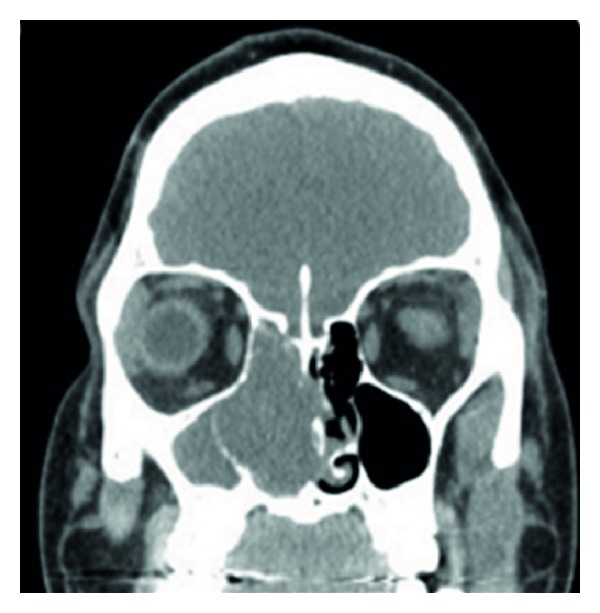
A noncontrast head CT revealing an LCH extending from the right maxillary and ethmoid sinuses to the right nasal choana.
